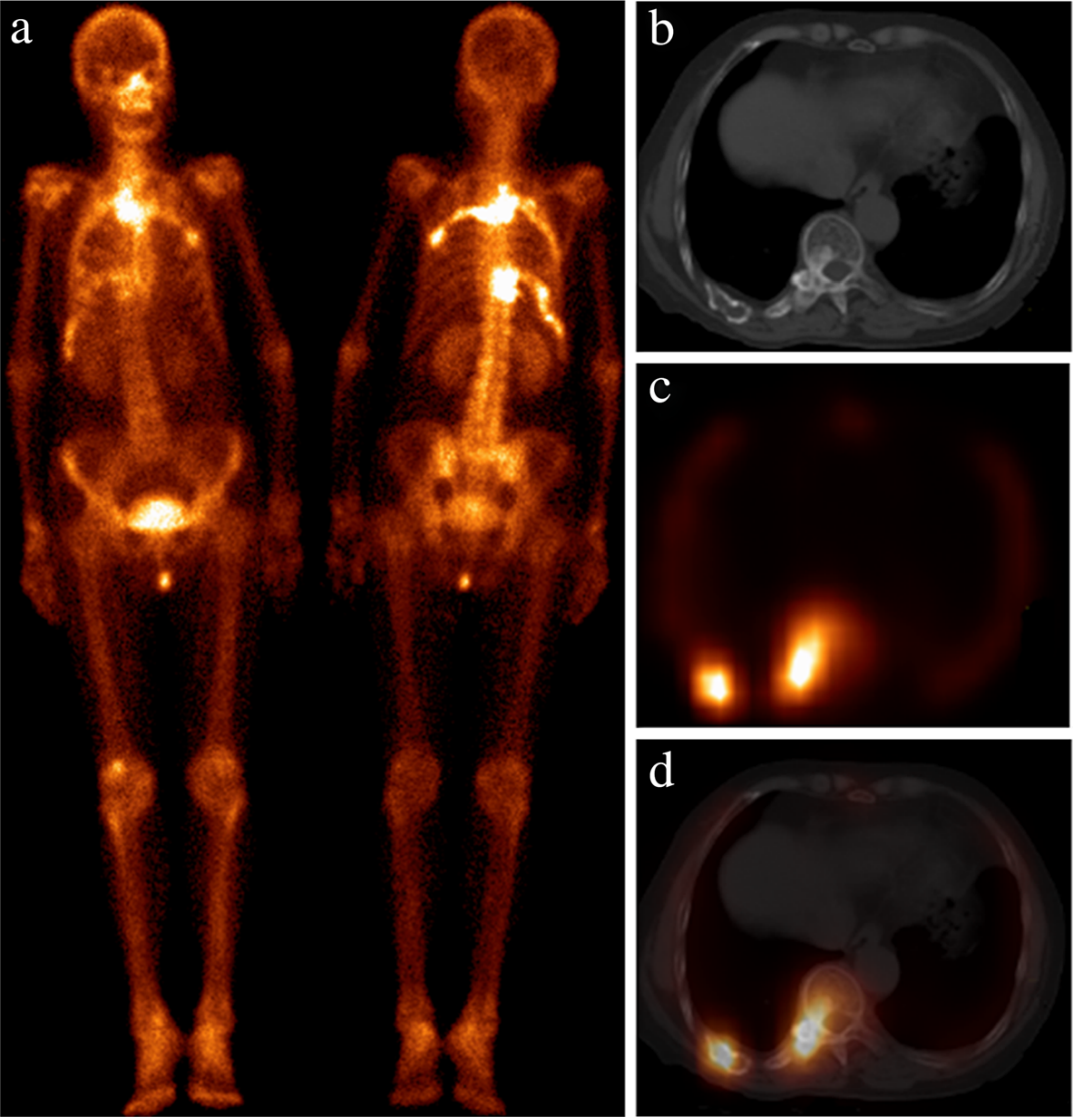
Patient 14 presented with HCC. On the WBS (a), there were multiple areas of abnormal ^99m^Tc-MDP uptake seen in the bilateral ribs and thoracic vertebra, which may mimic multiple bone metastasise. Axial CT (b), SPECT (c), and hybrid SPECT/spiral CT imaging (d) depicted increasing ^99m^Tc-MDP uptake corresponding to an expansile and lytic lesion with GGO in the lesion of bilateral ribs and thoracic vertebra. During the 2-year follow-up, no difference was detected in the WBS and CT image. The diagnosis of FD was established by a combined assessment of clinical and radiologic follow-up
